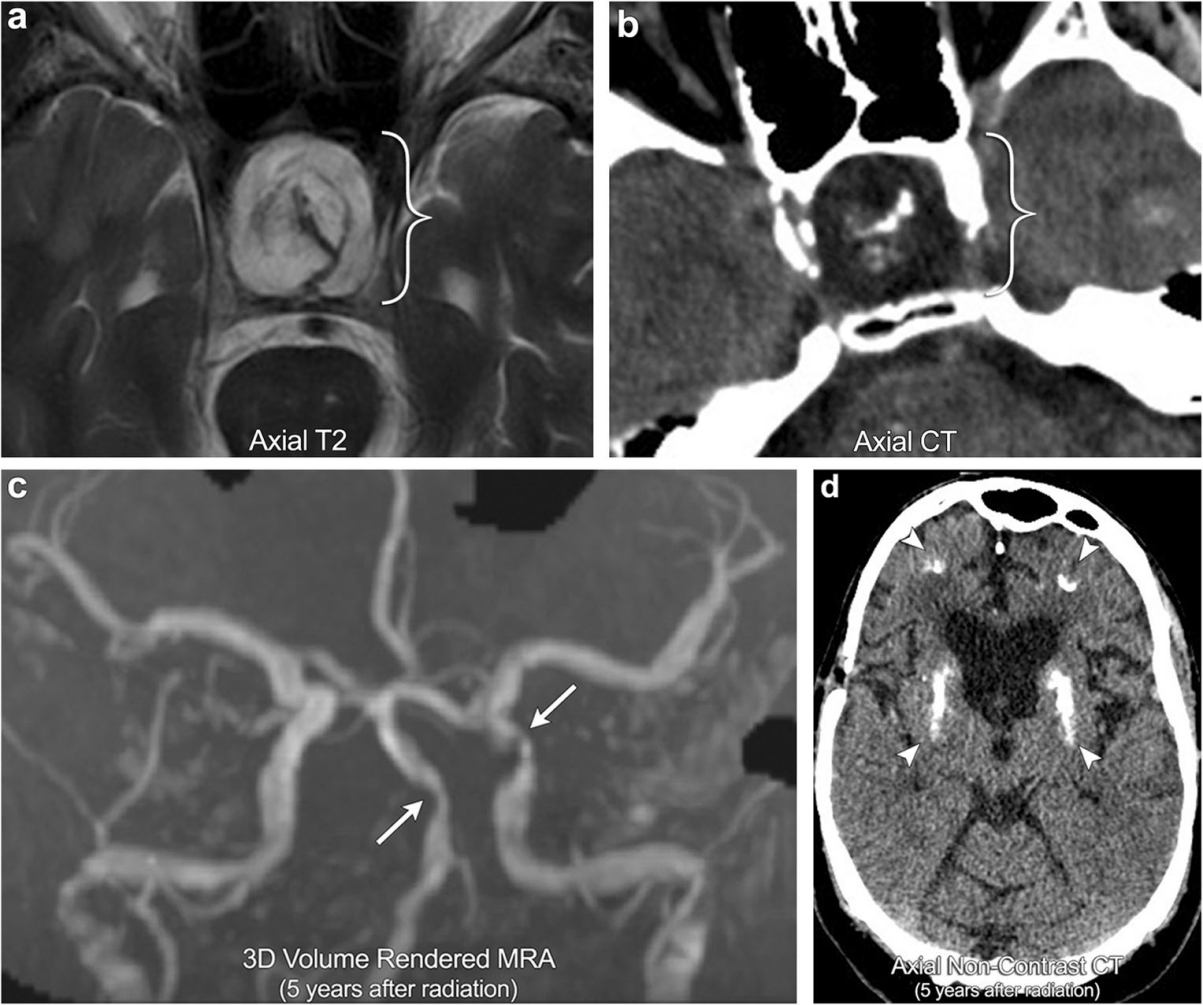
Radiation-induced vasculopathy and mineralising microangiopathy. A 35-year-old male with a history of radiation for craniopharyngioma (brackets). The patient went on to develop multiple strokes over subsequent years with MRA images demonstrating multiple areas of severe stenosis of the intracranial vasculature, most notable in the basilar artery and left supraclinoid ICA (arrows); this is compatible with radiation-induced vasculopathy. CT demonstrates dense calcifications in the subcortical white matter and basal ganglia (arrowheads), consistent with mineralising microangiopathy
